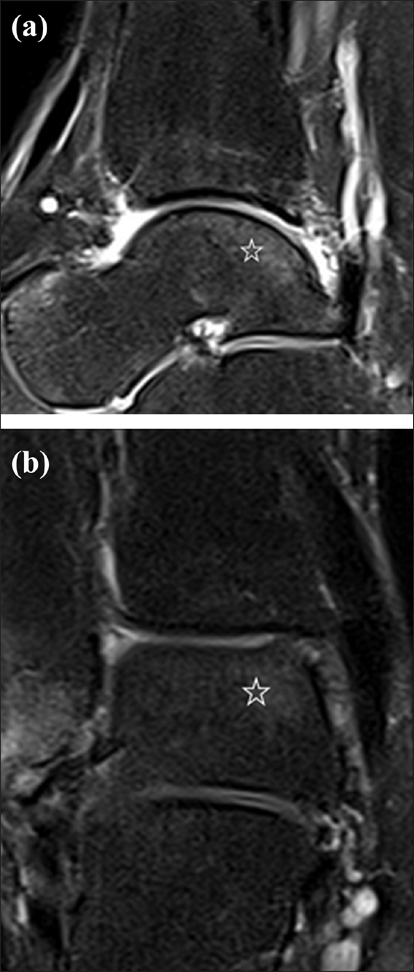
Stage 1 lesion according to Anderson classification. Sagittal PD fat suppressed MRI image (a) showing BME (star) at the posteromedial part of the talar dome. Coronal PD fat suppressed MRI image (b) revealing BME (star) in the posteromedial part of the talar dome.
